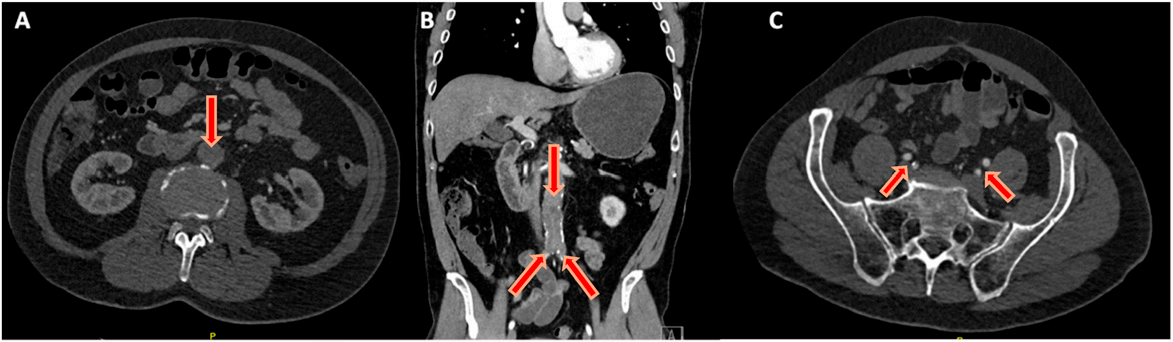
Representative cuts from a CT angiogram demonstrating complete occlusion of the aortobiiliac graft. (A) Axial image demonstrating a lack of contrast within the infrarenal aorta. (B) Coronal view demonstrating opacification of the ascending aorta (AA), Superior mesenteric artery (SMA) and left renal artery (LRA). Note the lack of contrast within the infrarenal graft. (C) Axial view demonstrating reconstitution of luminal flow in the external and internal iliac arteries via collaterals.
